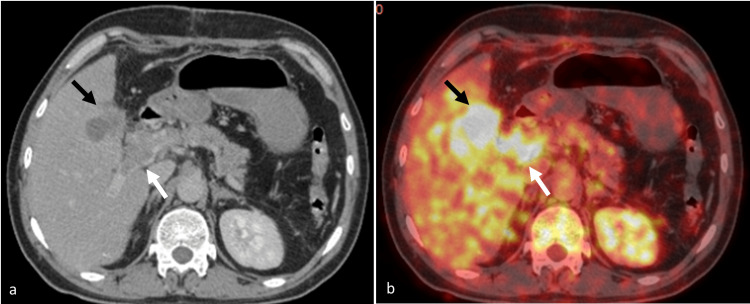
PET-CT showing the gall bladder mass and the tumor thrombus: (a) axial contrast-enhanced CT image shows heterogeneously enhancing irregular mass in the gall bladder fossa (black arrow), infiltrating segment 5 of the liver and surrounding fat stranding. An enhancing filling defect is seen in the main portal vein (white arrow), with expansion of the lumen, suggestive of tumor thrombus. (b) The corresponding PET-CT image shows uptake in the mass in the gall bladder fossa (black arrow) and the tumor thrombus (white arrow)
